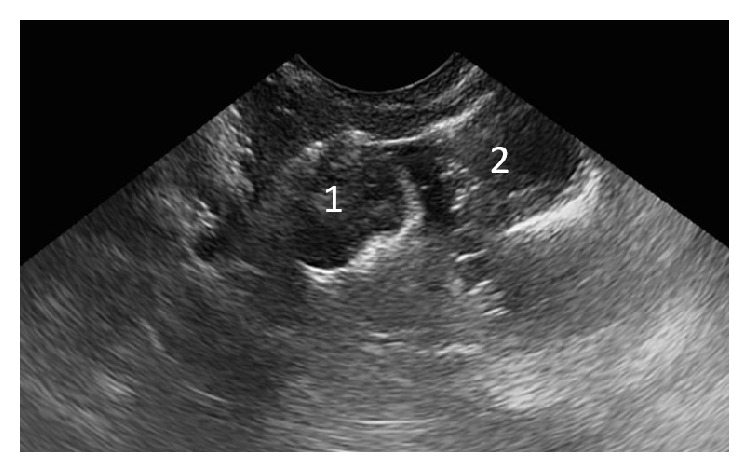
Findings in transabdominal ultrasonography. Each Foley catheter balloon (1) was inflated with normal saline to fix the catheter in the proximal vagina. About 30 ml of saline was injected through the catheters and the proximal vaginal pouch (2) was distended with saline.
